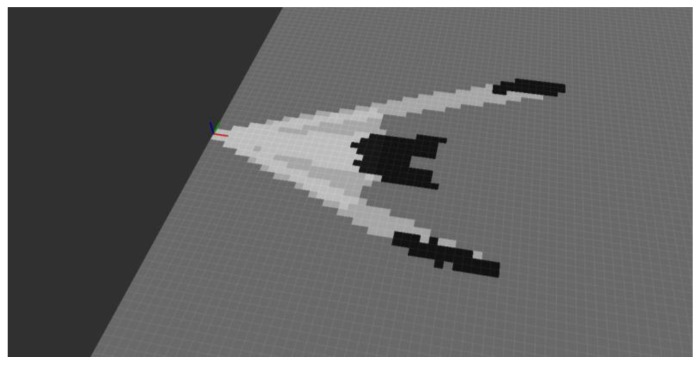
Occupancy grid computed from both the ultrasound and the depth camera measurements.
